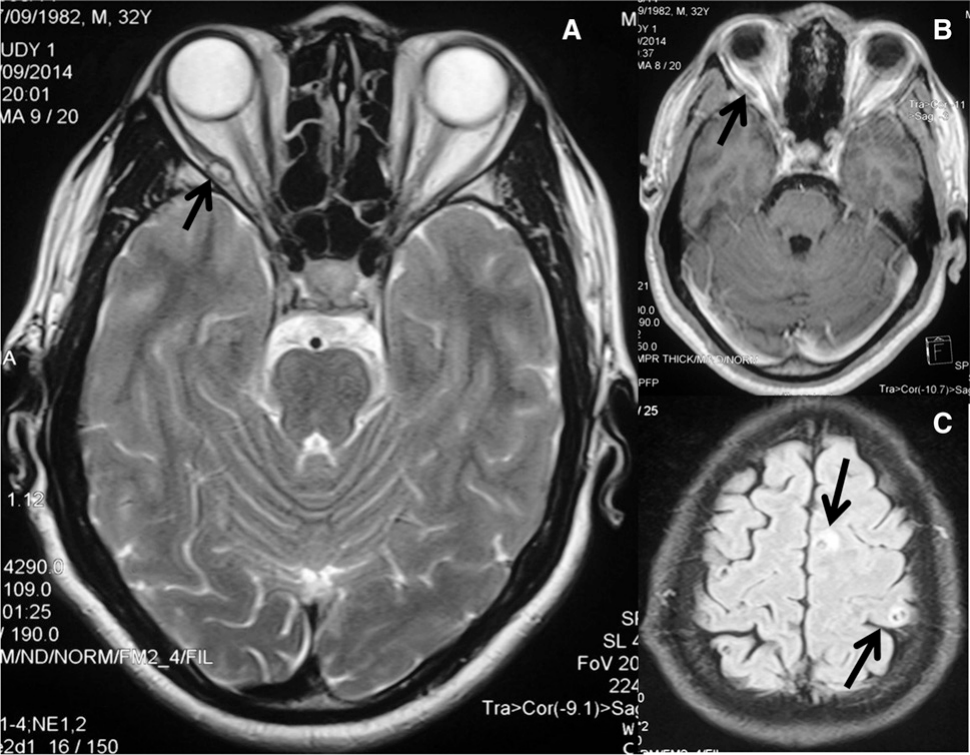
(A) T2W cranial magnetic resonance imaging (MRI) showing cystic lesion in the right lateral rectus with an eccentric scolex. (B) Contrast-enhanced cranial MRI showing minimal patchy enhancement of the lesion the right lateral rectus. (C) T2W cranial MRI showing multiple cysts with scolices and perilesional edema at high frontal brain parenchyma.
